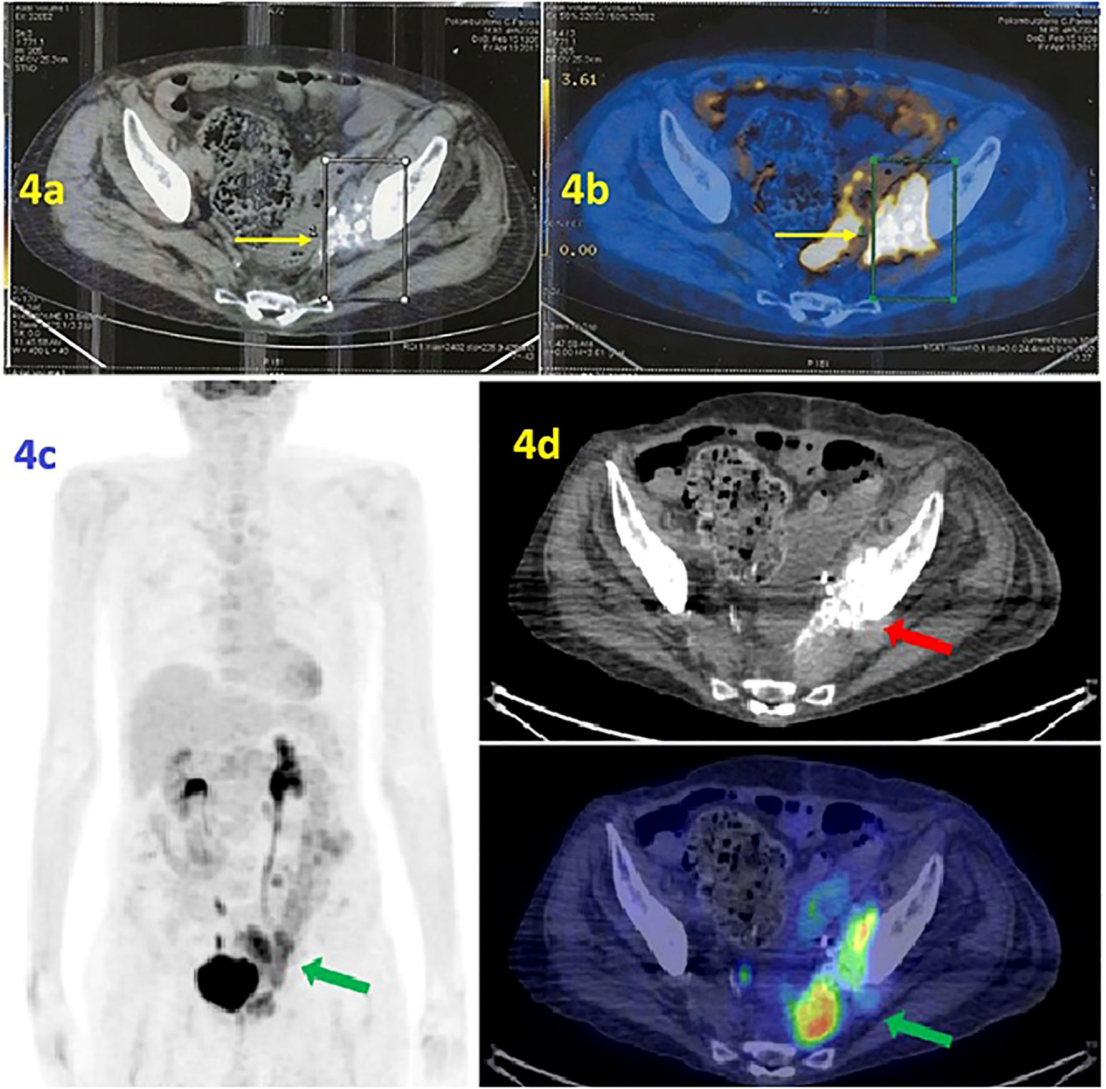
(a, b) PET scan/CT scan. (a) The PET scan (performed at IRCCS *Casa Sollievo della Sofferenza* Foundation in April 2017) shows radiopharmaceutical hyperaccumulation in the left area of the pelvis with an mSUV of 10.1. (b) Co-registration CT scan documented the volumetric increase of lesion. Note the psammomatous bodies, a typical marker of ovarian tumors such as adenocarcinoma of Mullerian origin. (c, d) [18F] FDG PET/CT MIP reconstruction and transaxial images (performed at the University Hospital of Bari in June 2019) showed the presence of a tumor lesion in the area near the left side of the bladder, adjacent to the obturator muscle and extended anteriorly to the bladder with an mSUV of 12.5 (green arrow). The psammomatous bodies are evident, a marker of ovarian tumors that confirms the tumor’s Mullerian origin (red arrow).
